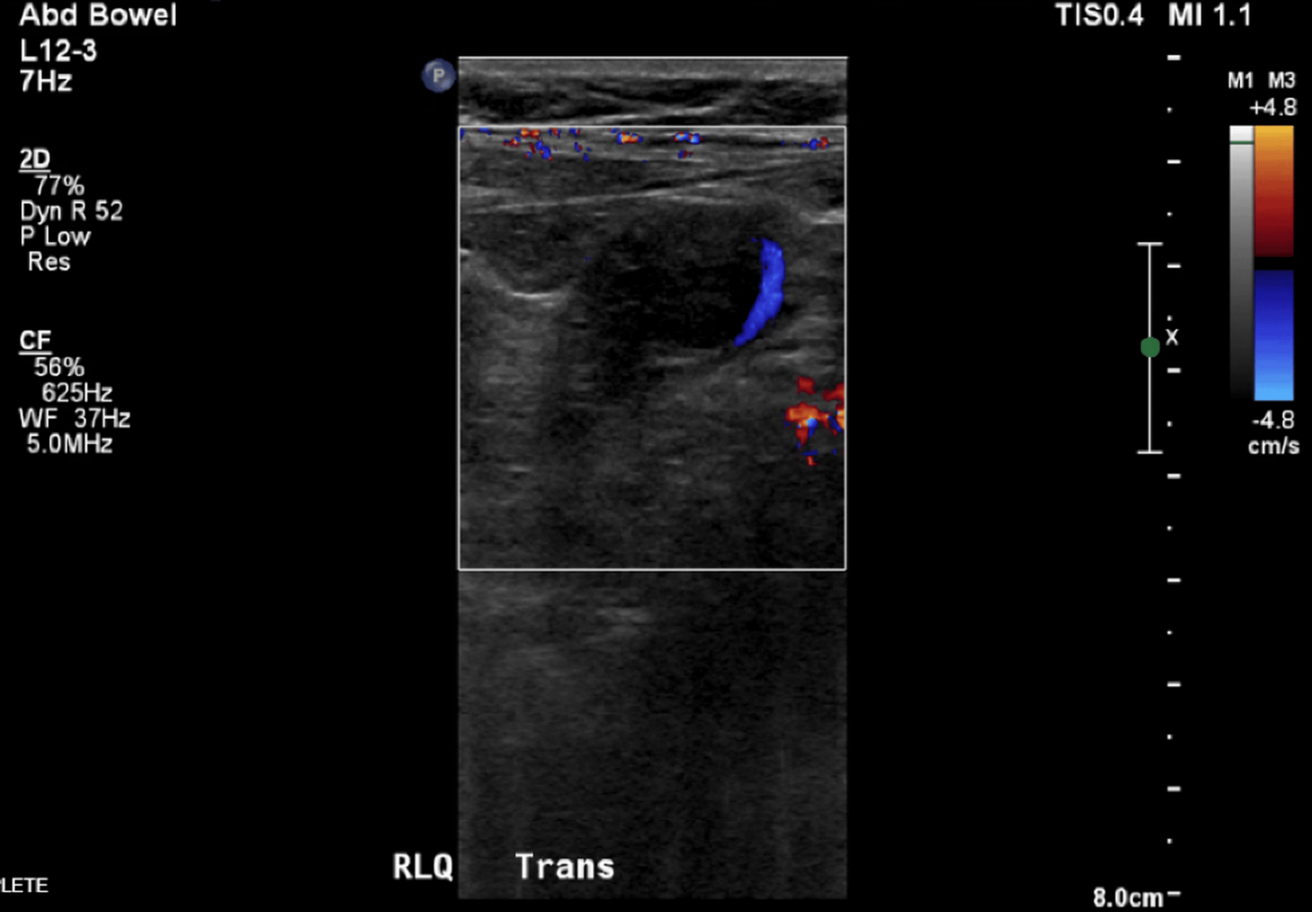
Ultrasound image showing a tubular hollow viscus structure in the right lower quadrant, raising concern for appendiceal or bowel pathology.
